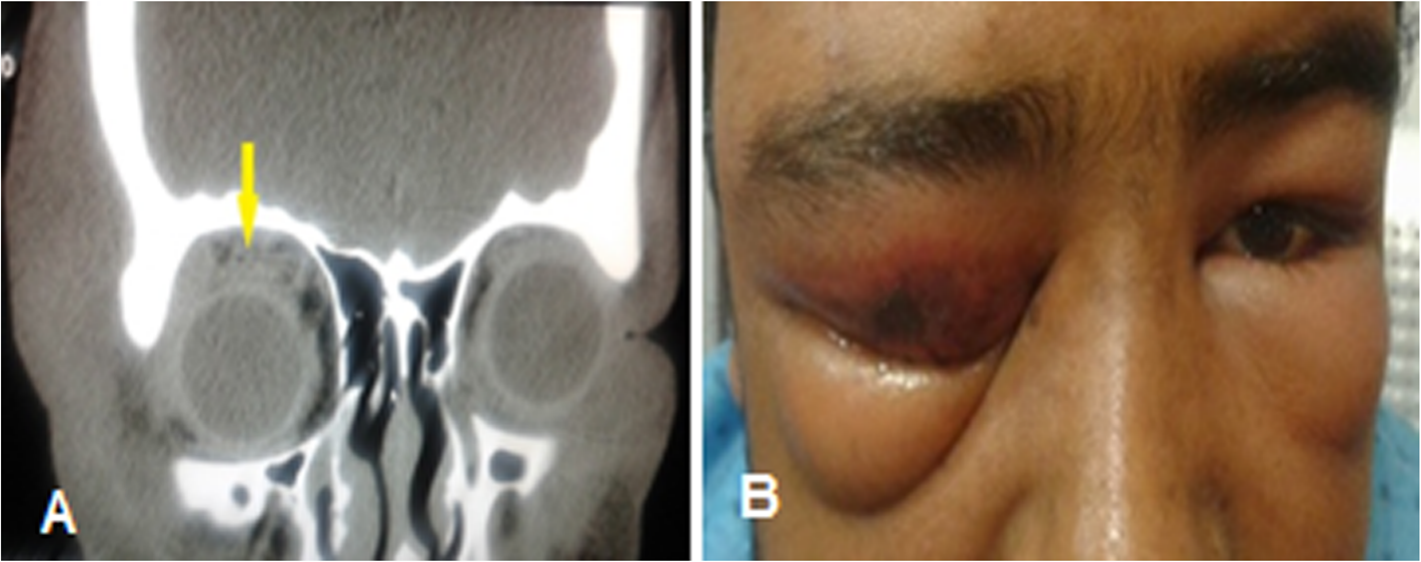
Superior orbital cellulitis due to unknown insect bite that was managed with intravenous antibiotics without any surgical intervention, a external eye examination shows eyelids edema and upper lid redness with a small skin necrosis in site of the bite, b Orbital CT scan coronal view revealed superior orbital infiltration without abscess formation
